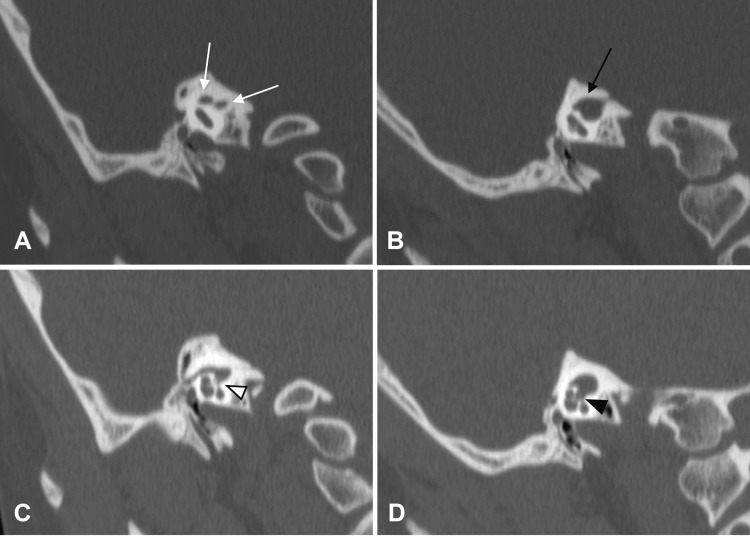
CT of the temporal bones: the right temporal bone in Pöschl view demonstrates (A) a double-barreled IAC (white arrows) and (C) poor communication with the cochlea through the cochlear aperture (white arrowhead). The left temporal bone in Pöschl view shows (B) a single, patent IAC (black arrow) and (D) clear communication with the cochlea via a patent cochlear aperture (black arrowhead) CT: computed tomography; IAC: internal auditory canal
